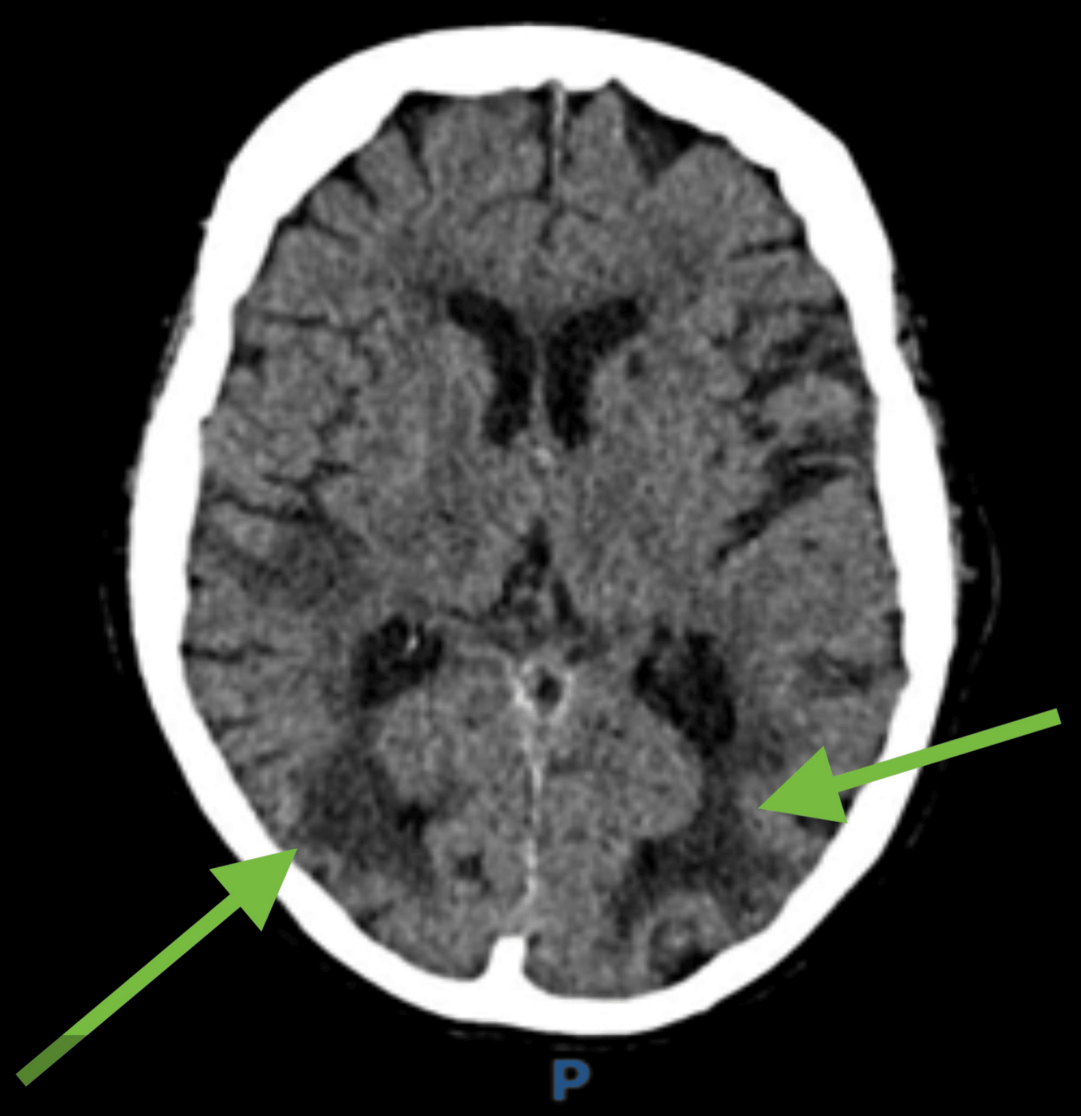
Non-contrast CT brain imaging revealed bilateral occipital and posterior parietal hypodensities (green arrow).
